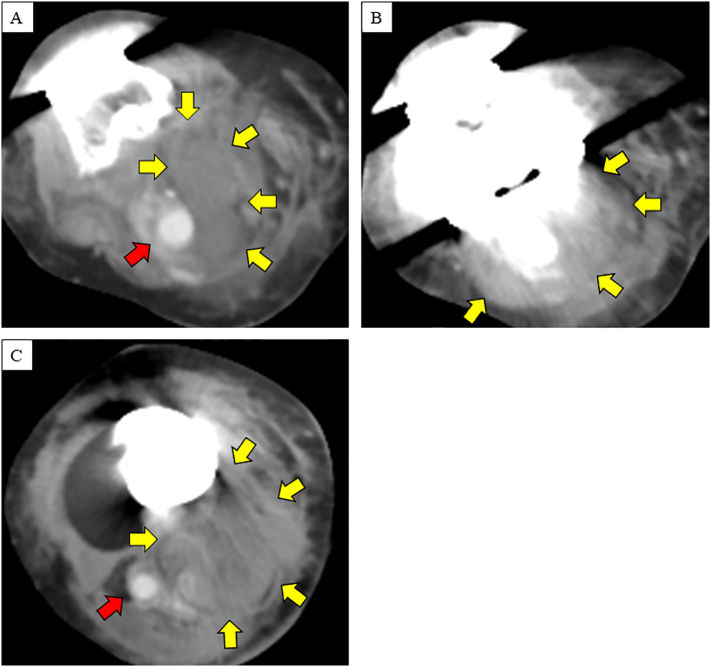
Enhanced computed tomography performed at the previous hospital. Right popliteal artery is denoted by the red arrow and hematoma by the yellow arrows. (A) The hematoma was close to the popliteal aneurysm above the right knee. (B) The image artifact caused by the right artificial knee joint interfered with detailed evaluation. (C) The massive hematoma in the posterior segment of the right lower limb below the knee.
